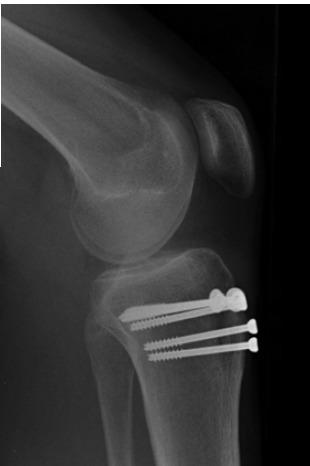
Postoperative lateral radiograph of the left knee at 24
